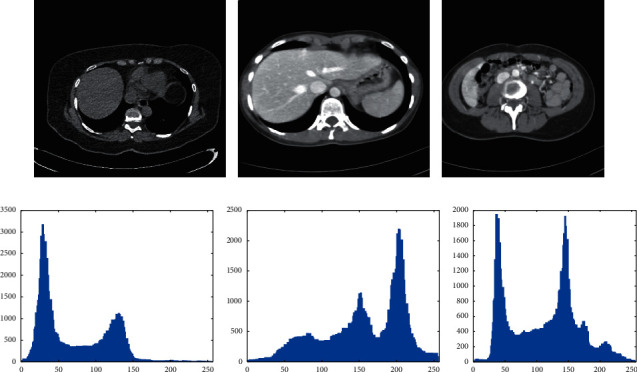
Upper, middle, and lower slices of the liver CT image and their histograms. (a) The upper slice. (b) The middle slice. (c) The lower slice. (d) Gray-level histogram of (a). (e) Gray-level histogram of (b). (f) Gray-level histogram of (c).
